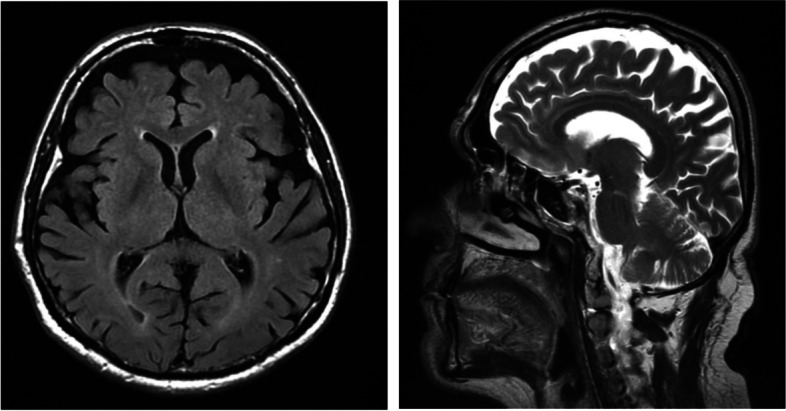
MRI of the head in the T1 sequence without contrast (A) revealed supratentorial and infratentorial cortical atrophy, retrograde vascular lesion (Fazakes scale: grade 1) and in the T2 sequence (B) without contrast revealed partial empty sella syndrome
